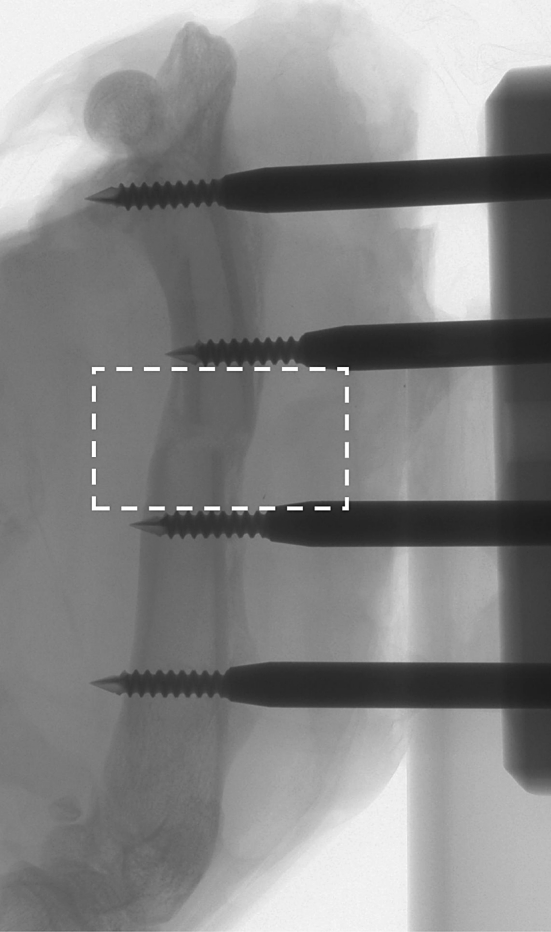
Radiograph of healed fracture (and fixator) sacrificed 6 weeks after fracture. The fracture zone is outlined
